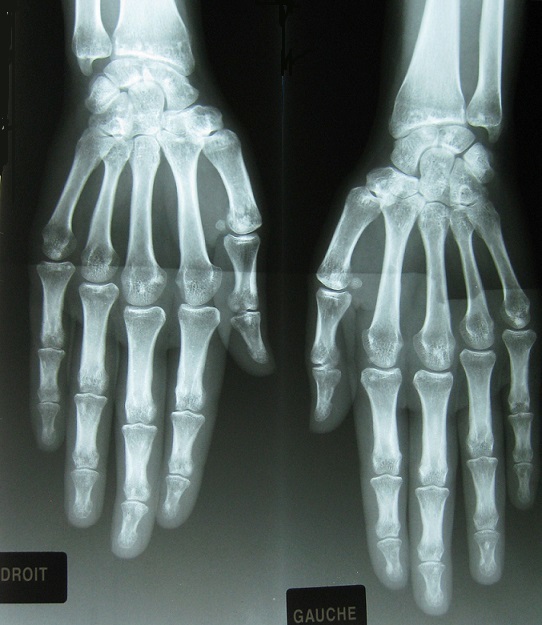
Radiographie des mains de face montrant des lésions denses intéressant les os du carpe, l’extrémité inférieure du radius et du cubitus bilatérales et symétriques en rapport avec une ostéopoécilie
